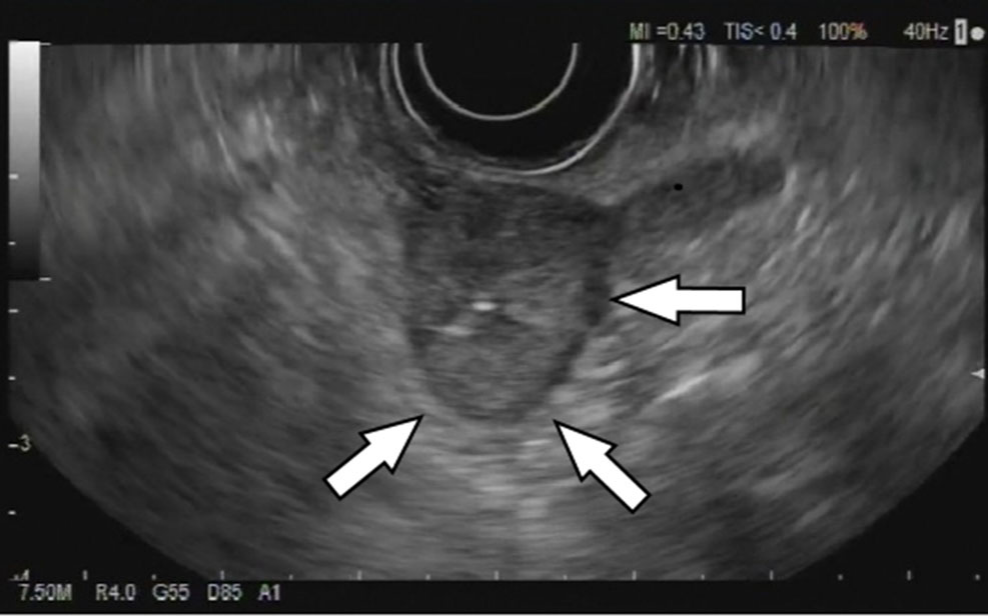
Endoscopic ultrasound. Presence of a lesion rising from the IV layer of the stomach wall (*white arrows*)
